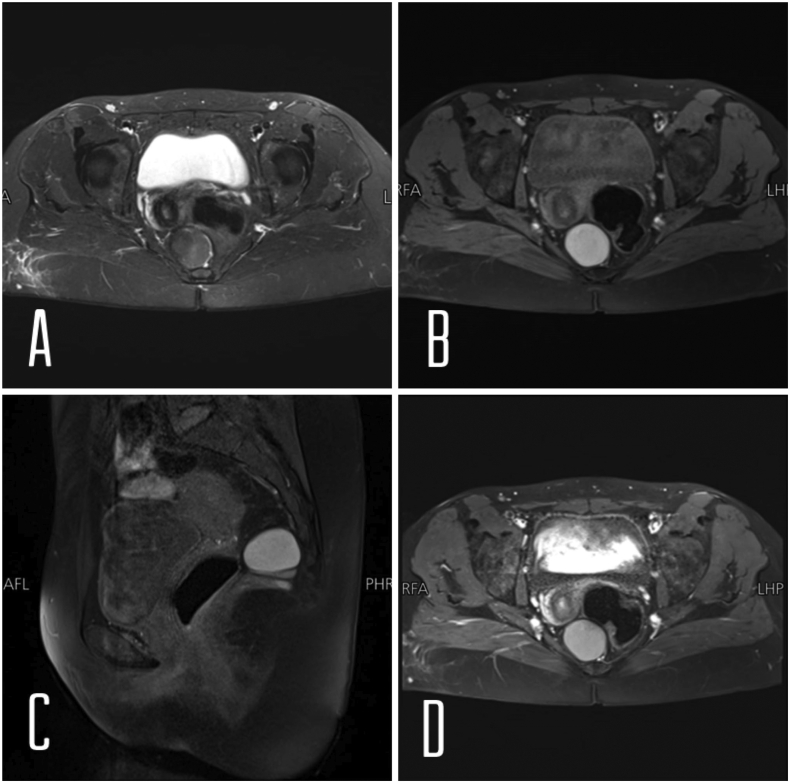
Magnetic resonance imaging (MRI) showed entirely capsulated cystic mass which was located in the right dorsal side of the rectum . The main bulk of the lesion appears hypo-intense on T2-weighted imaging (A), but homogeneously hyper-intense on T1-weighted imaging (B and C) with no significant enhancement post contrast administration (D).
